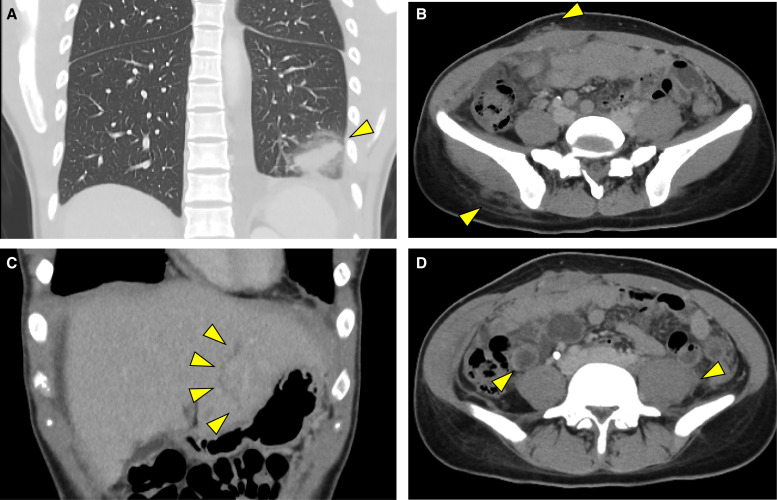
Computed tomography findings for the patient. (A) Club-shaped consolidations in the lower lobe of the left lung. (B) Subcutaneous nodular lesions in the abdominal wall and buttocks. (C) Funicular low-density area in the liver, indicating hepatic migration. (D) Enteritis and enlargement of the left iliopsoas muscle.
